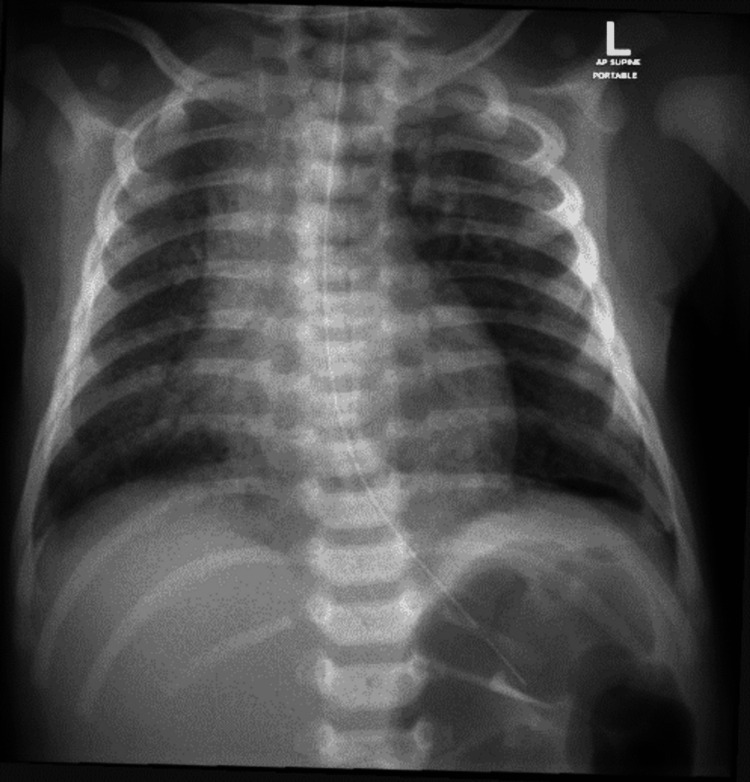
Chest X-ray 14 hours post LISA LISA: Less invasive surfactant administration
